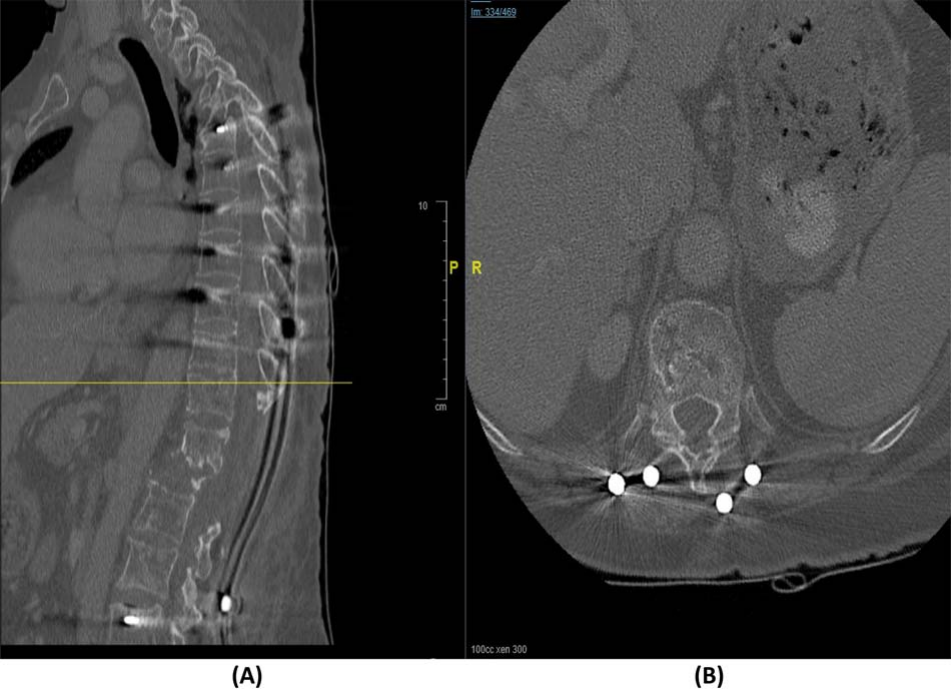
(A) Sagittal spine CT demonstrating healed bone and re-ossification of T11-L3. (B) Axial spine CT of the corresponding level of T11 vertebral body.
